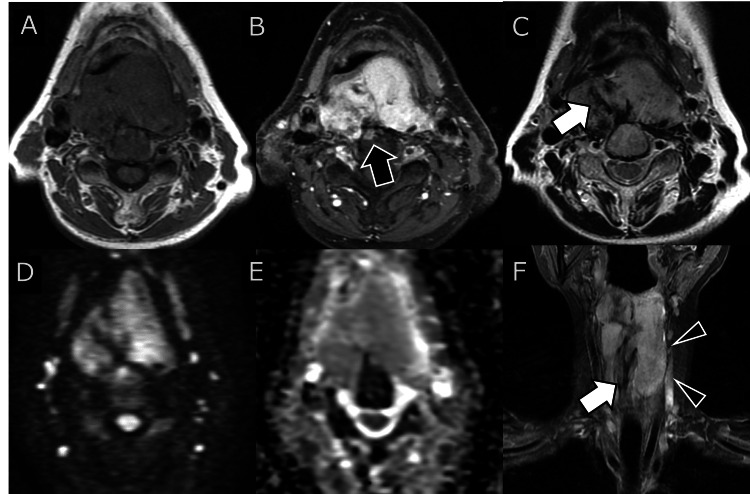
Magnetic resonance imaging (MRI) findings of the lesion. (A) T1-weighted image: The tumor demonstrates signal intensity comparable to or slightly lower than that of muscle.
